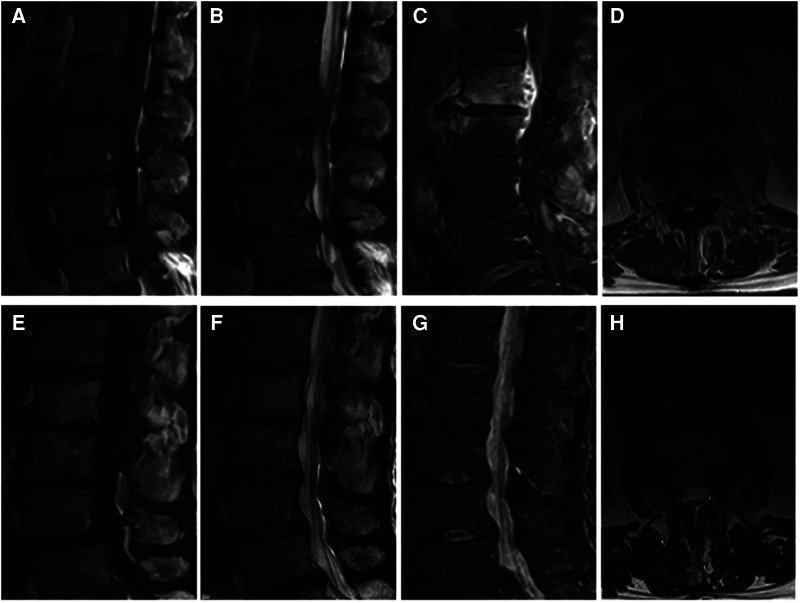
(A–D) It presents the MRI scans of patient No. 5. Sagittal and axial magnetic resonance images with L2-L3 intervertebral infection. (E–H) It presents the postoperative the MRI scans (9 months after surgery) of patient No. 5. MRI shows that the abscess was disappeared without recurrence. (A,E) Sagittal T1WI. (B,F) Sagittal T2WI. (C,G) Sagittal contrast-enhanced image. (D,H) Axial image after surgery.
